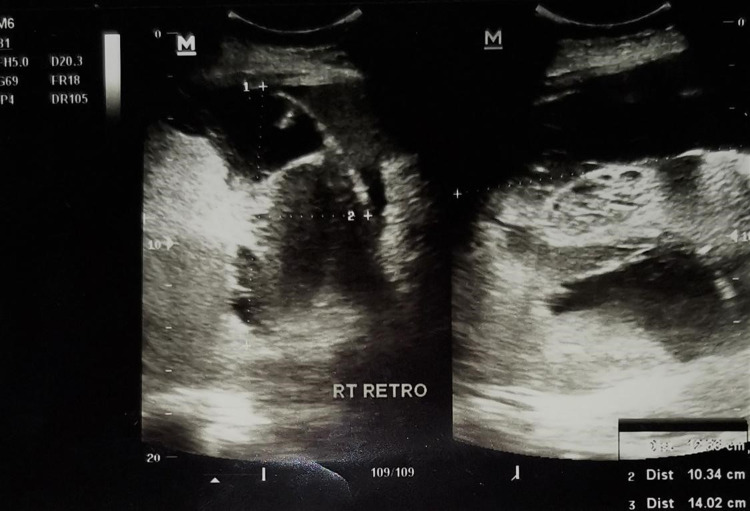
Abdominal ultrasonography showed a right retroperitoneal hematoma
